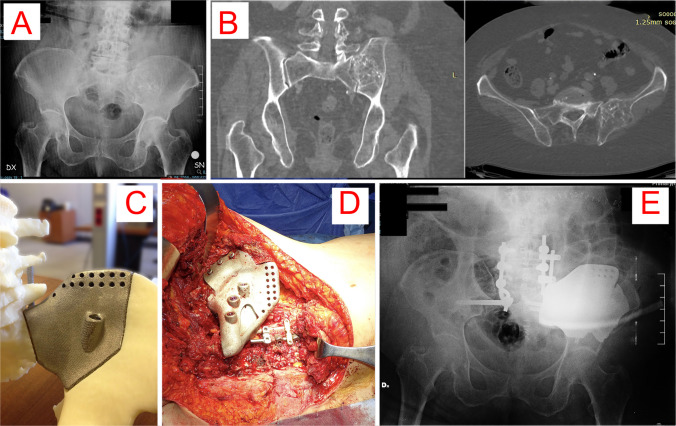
Seventy-three-year-old woman affected by grade 2 CS of the iliac side of the sacroiliac joint; in (A) the preoperative X-ray highlights the osseous rearrangement and calcifications more clearly seen in CT scan (B). In (C) a preoperative model of the custom-made prosthesis designed for reconstruction; in (D) an intraoperative photo of the reconstruction with the sacroiliac prosthesis
